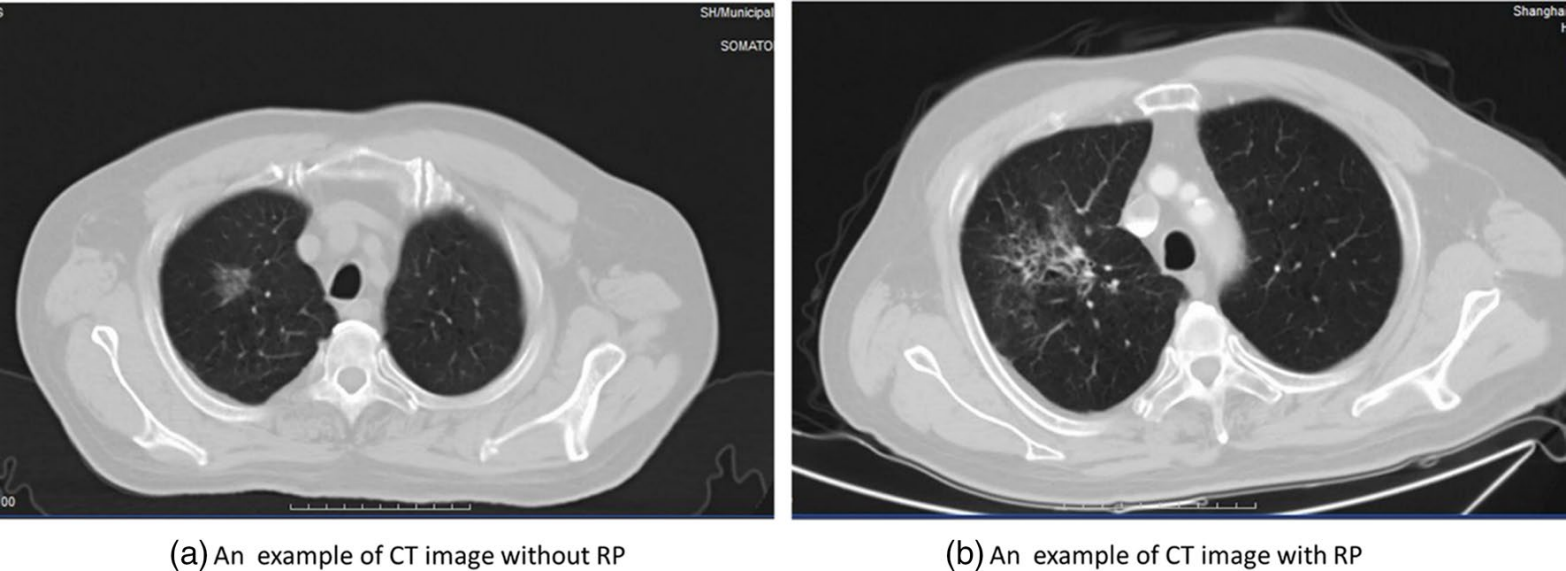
CT image examples of a CT without/with RP
